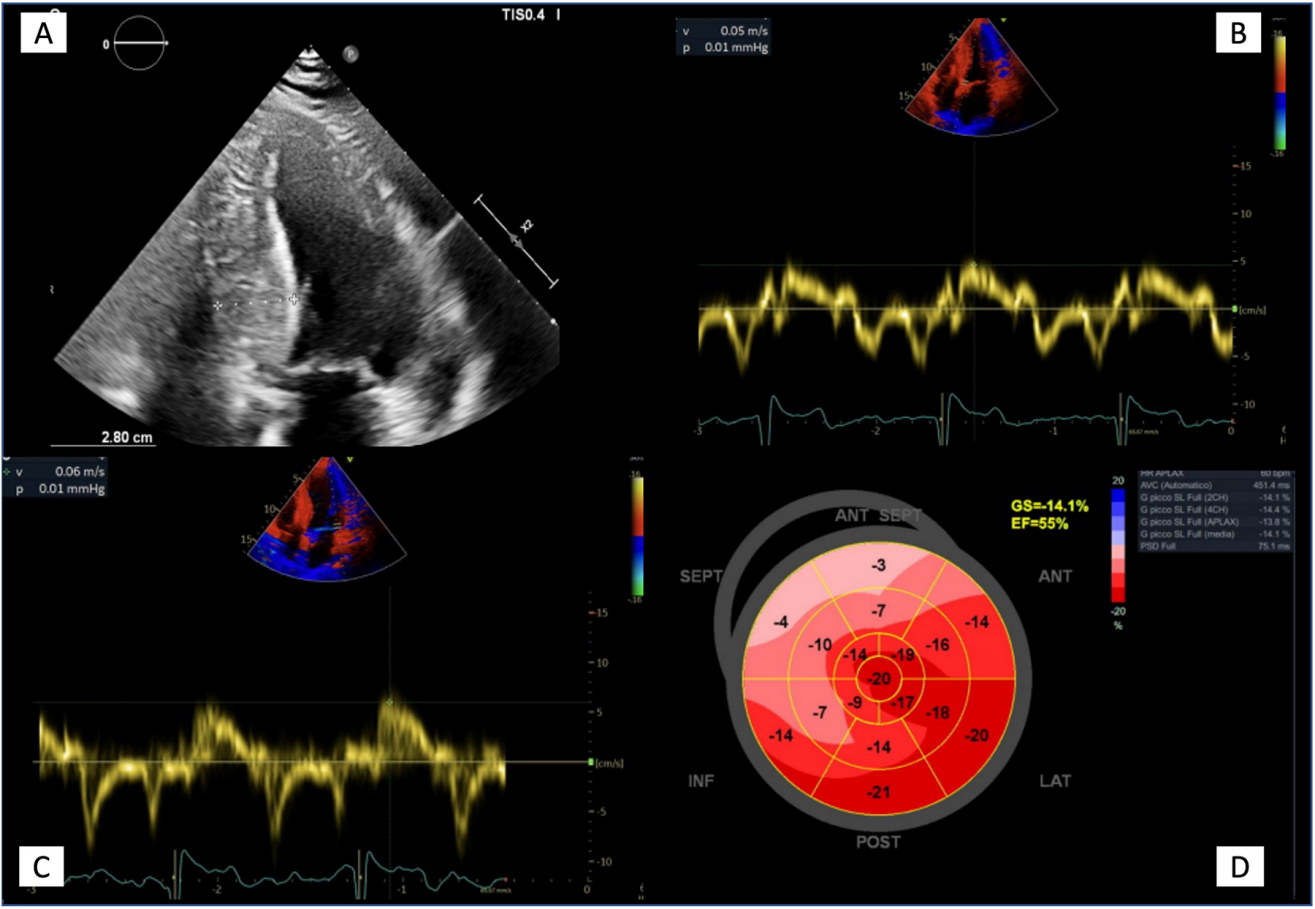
Echocardiographic features in advanced Anderson-Fabry disease cardiomyopathy. (A) Apical four chambers view showing the maximal wall thickness (28 mm) and the typical binary sign; (B, C) Tissue Doppler mitral annular velocities at septal and lateral corners respectively, showing low systolic velocities (5 and 6 cm/s respectively); (D) 2D speckle tracking analysis bull's eye plot, showing reduced LV GLS value (−14.1%).
